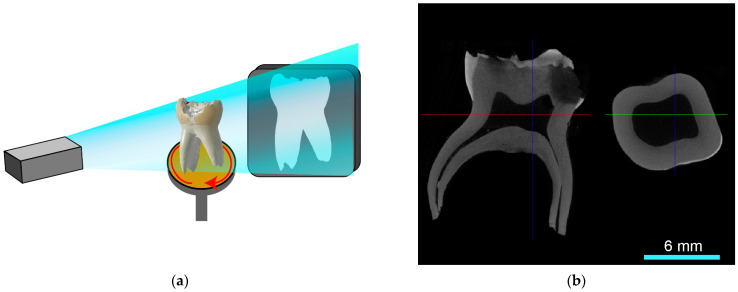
(a) In μ-CT measurements, probes are rotated. The electron beam is attenuated by the sample and presented on a detector panel. (b) Exemplary μ-CT reconstruction of a primary molar in coronal and transverse plane (scale of 6 mm). The right section of the image shows the transverse plane indicated by the red line.
